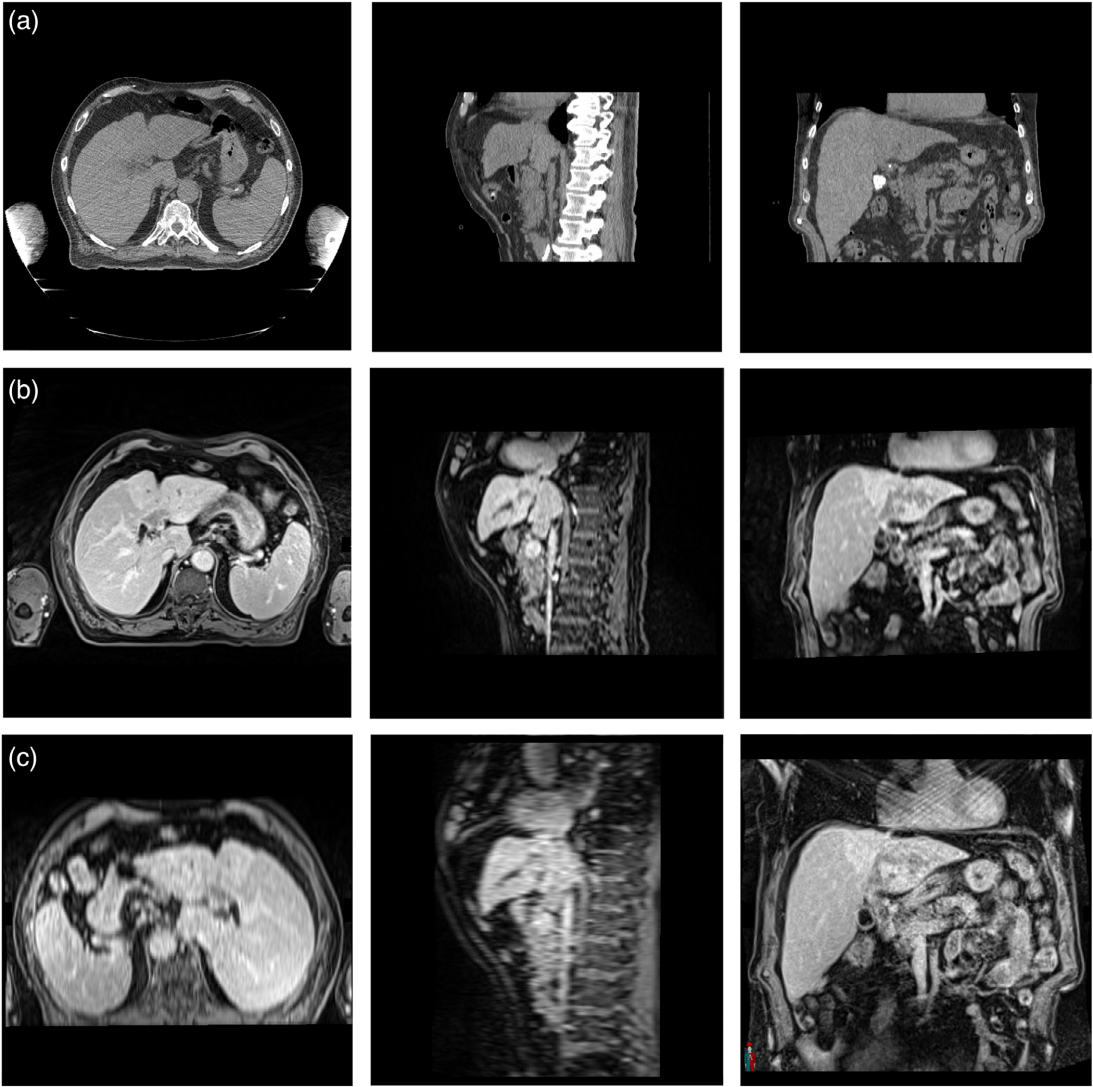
Model axial, sagittal, and coronal slices for (a) CT, (b) axial 5‐bin MR, and (c) coronal 10‐bin MR image sets for a compression‐belt patient with a liver lesion.
